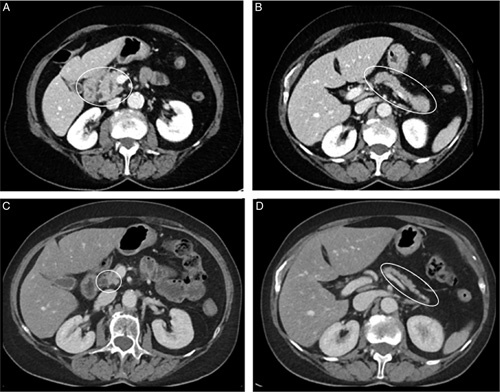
Follow-up CT of the pancreas. During presentation with abdominal pain and high lipase (A and B) CT showed an acute pancreatitis with and edematous head (A) and tail (B) of the pancreas. Five months later the pancreas appears to be atrophic (C; pancreatic head, D; pancreatic tail) without signs of inflammation.
